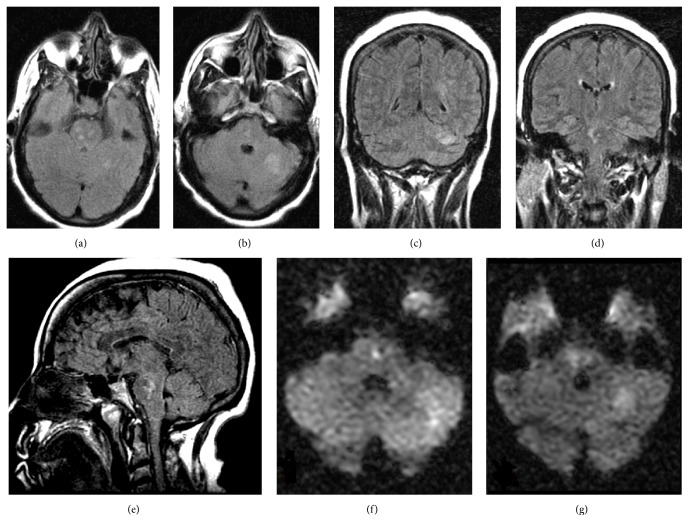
Brain MRI. (a, b, c) Abnormal FLAIR signal involving the left cerebellum. (a, b, d, e) Abnormal FLAIR signal involving several small foci within the pons. (f and g) Diffusion weighted imaging sequence consistent with multiple regions of acute ischemia in the pons and left cerebellum.
